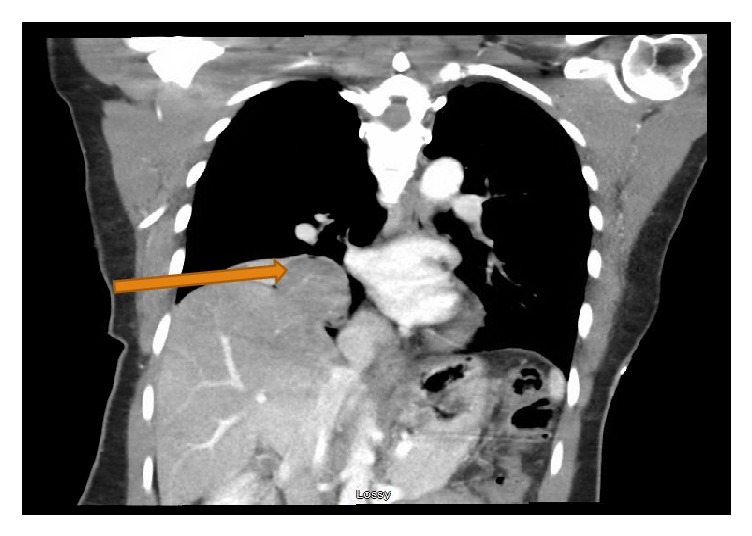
Coronal view of CT scan with orange arrow showing a 33 mm diaphragmatic defect with liver herniation, 5.8 x 4.6 x 3.9cm.
